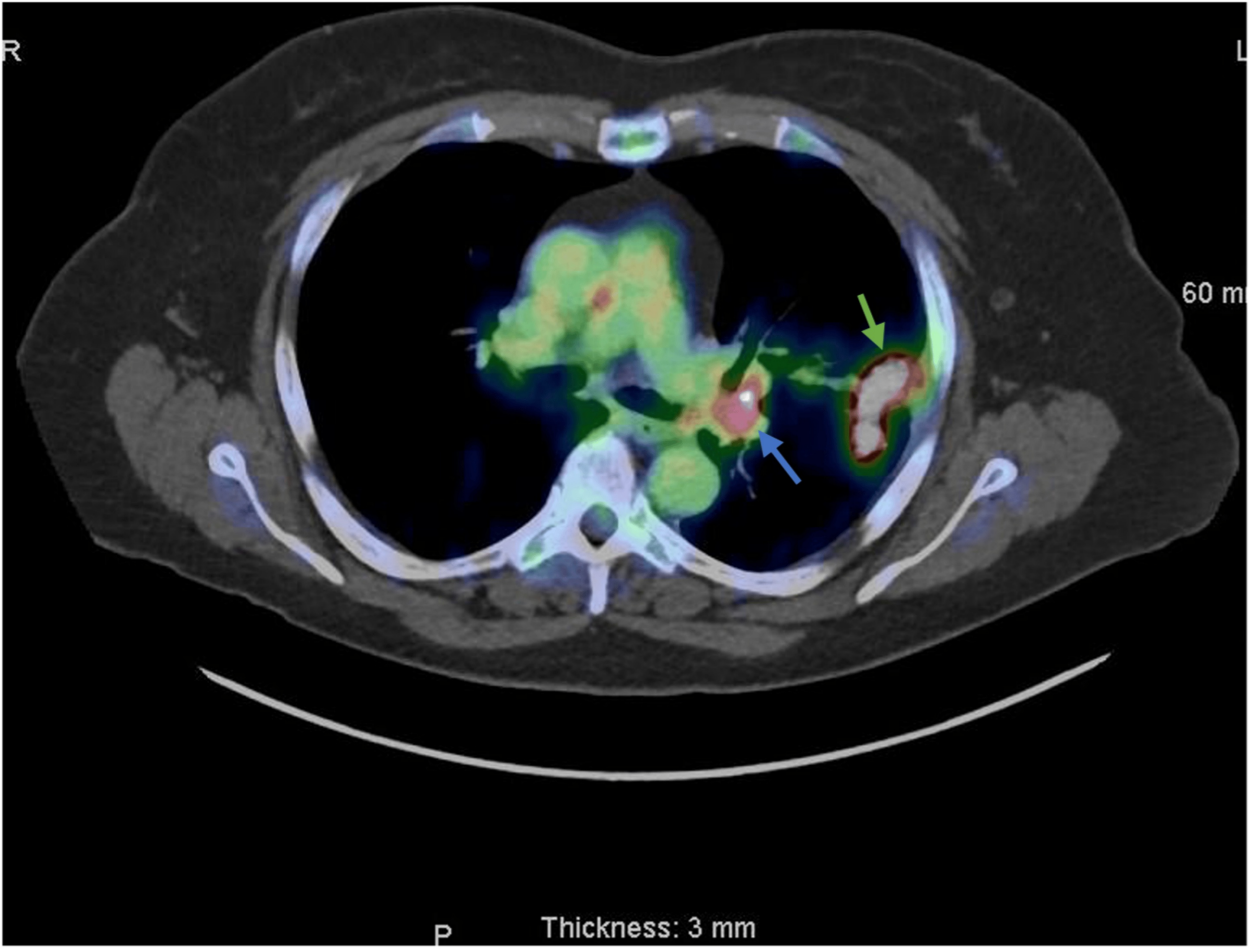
Axial view PET/CT revealing the FDG avidity of the left upper lobe (LUL) nodule (green arrow) and the ipsilateral mediastinal lymph nodes (blue arrow) The resolution of the pleural effusion and the decreasing size of the primary LUL nodule and mass are noted. Slice thickness: 3mm. Smooth reconstruction algorithm. PET/CT: positron emission tomography/computed tomography; FDG: fluorodeoxyglucose
